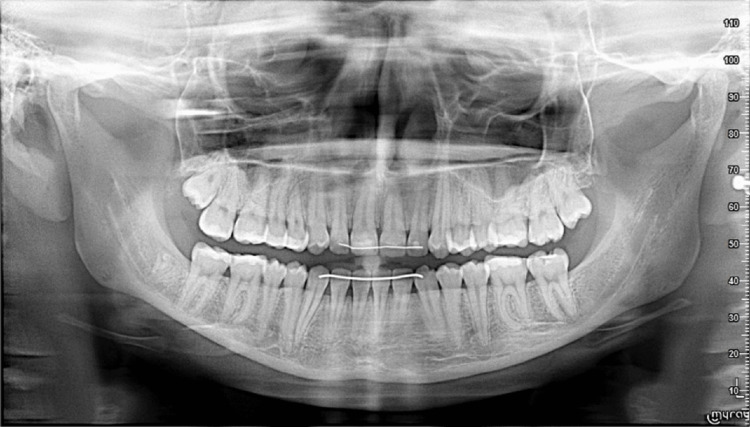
Post-treatment panoramic radiograph Panoramic radiograph taken after completion of orthodontic treatment, showing well‑aligned maxillary and mandibular arches, good root parallelism, and no evident root resorption or other radiographic abnormalities.
